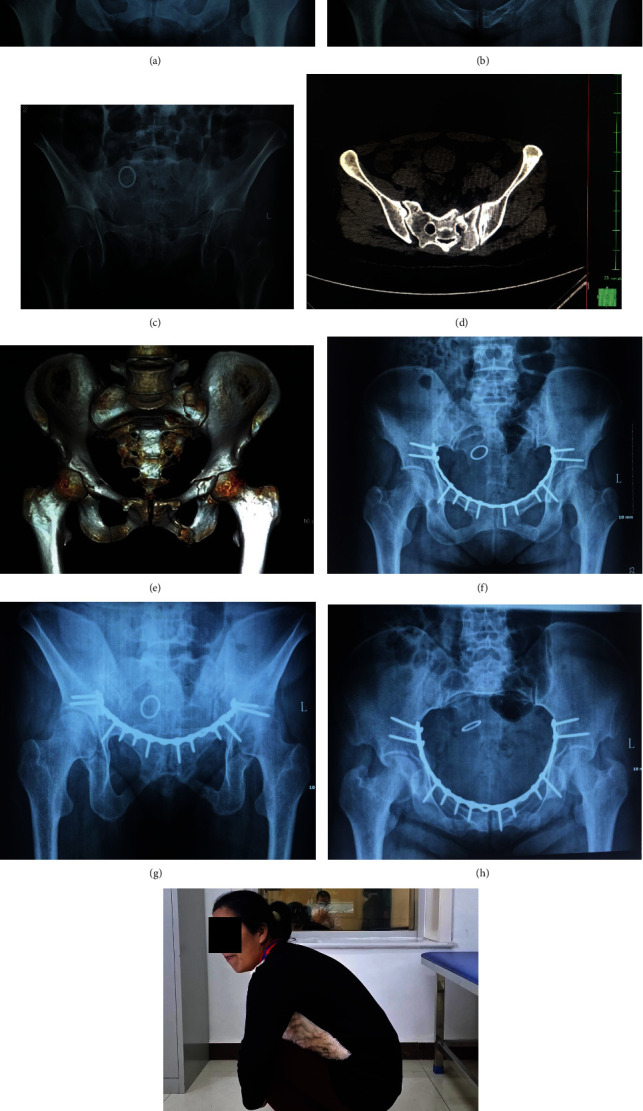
A 43-year-old female presenting with pelvic ring fractures (Young and Burgess LC-1/Tile B2). Preoperative AP view (a), inlet view(b), and outlet view(c) showed both pubic rami and a transforaminal sacral fracture on the left side. The CT scan (d) and 3D-reconstruction (e) showed the sagittal fracture line across the anterior and posterior cortex of S2 with minimal displacement. The pubic rami fractures were fixed through modified Stoppa approach. At final follow-up, the radiographs (f–h) and functional photographs (i) showed that the fractures healed, and the function recovered well.
